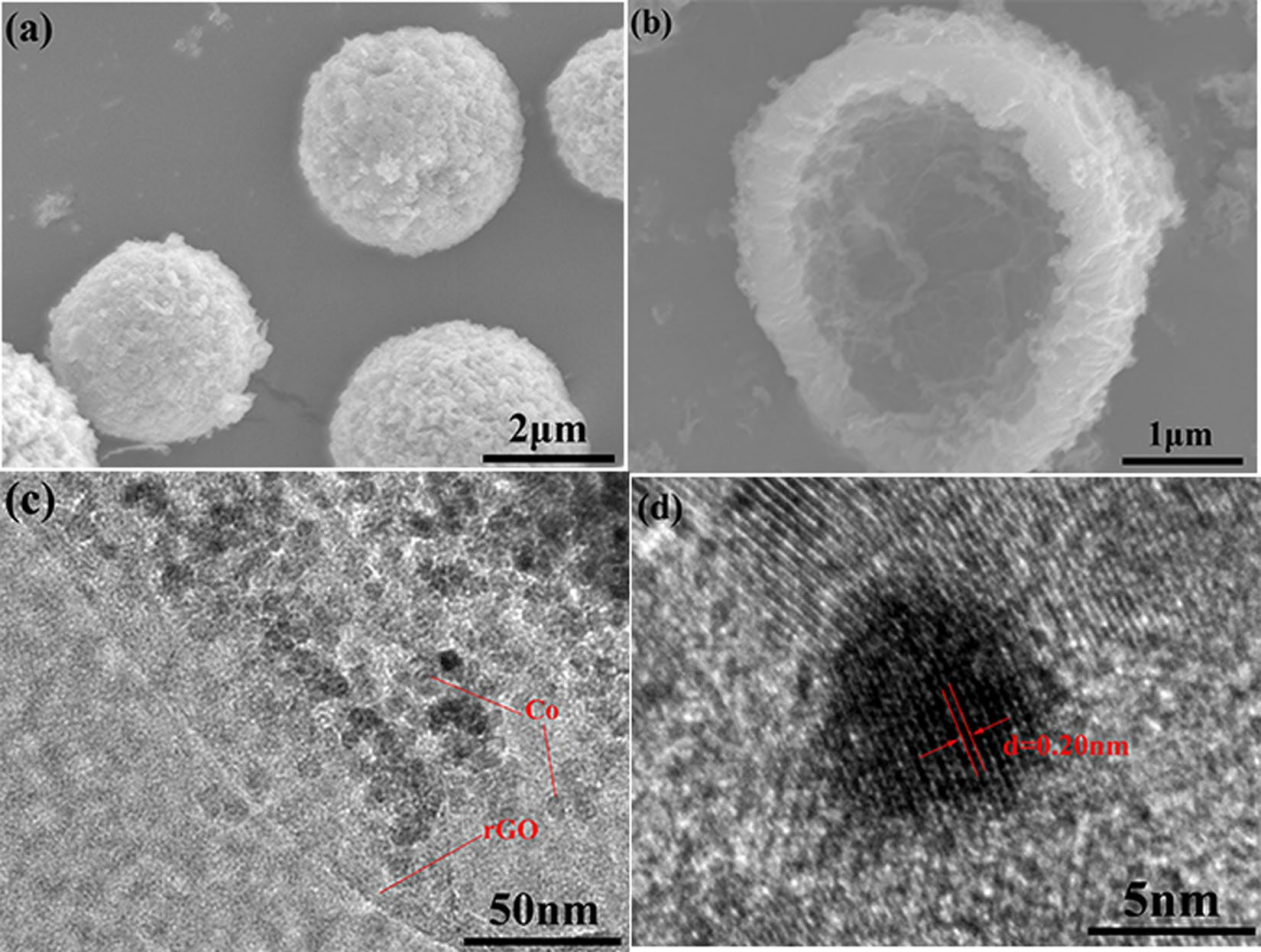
SEM images of (a) Air@rGO€Co microspheres (S_1.5_), (b) cross-section of microsphere. (c) TEM image of a fragment from a microsphere which has been ultrasonic decomposed, (d) high magnification TEM image of the fragment.
